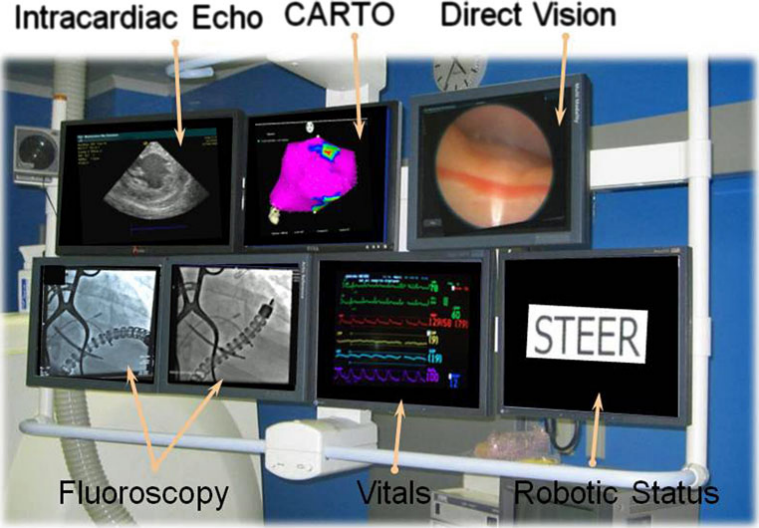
Monitors used during the clinical trial procedure. Information displayed include intracardiac echocardiogram, CARTO, fiberscope vision, fluoroscopy (2 monitors), vitals, and robotic status information. Images shown have been superimposed on the monitor bank
